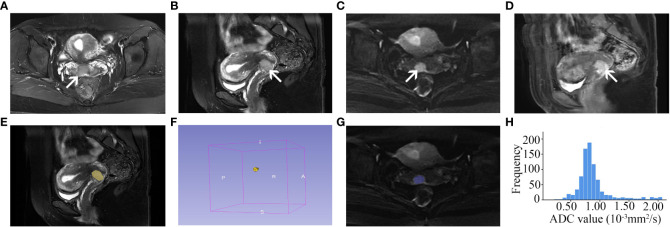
A 44-year-old cervical cancer patient with LVSI (FIGO stage IIA). Axial (A) and sagittal (B) FS T2-weighted image shows a tumor with slightly high signal intensity in the anterior lip of the cervix (arrow). The tumor is seen as high signal intensity on the axial diffusion-weighted image (C, arrow) and homogeneous enhancement on the contrast-enhanced image (D, arrow). The tumor shape is drawn on each contiguous tumor section of sagittal FS T2-weighted images to measure the tumor volume (E, F, yellow shadow). The VOI of the tumor is drawn on each contiguous tumor section of axial diffusion-weighted images (b = 800 s/mm^2^) (G, blue shadow), and the ADC histogram (H) is obtained based on the VOI. LVSI, lymphovascular space invasion; FIGO, International Federation of Obstetrics and Gynecology; FS, fat suppression; VOI, volume of interest; ADC, apparent diffusion coefficient.
